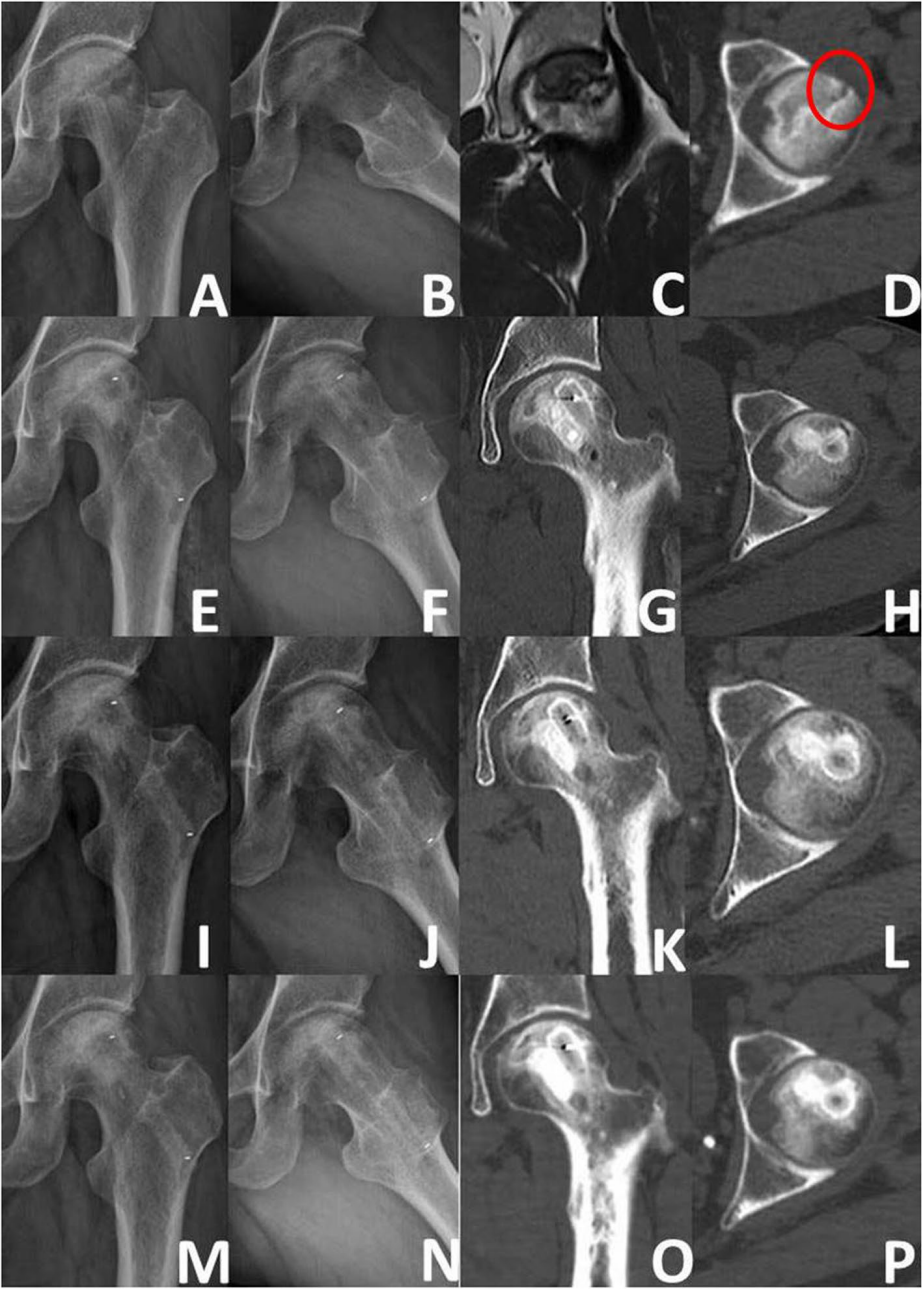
Representative case of ARCO IIIA. A, B Preoperative radiograph. C Preoperative MRI. D Preoperative CT (the red circle-subchondral fracture). E-H 3days post-surgery (X-ray and CT). I-L 3months post-surgery (X-ray and CT). M-P 36 months post-surgery (X-ray and CT)
